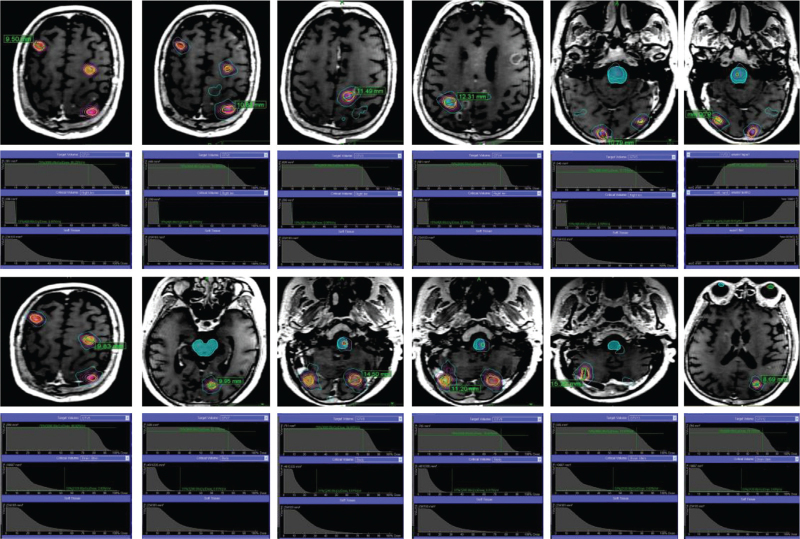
The location of tumors for the second cyberknife radiotherapy from positioning CT images and the excellent dose distribution of each tumor.
